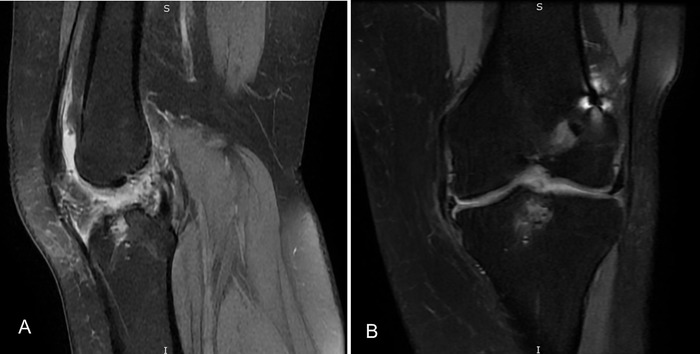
Magnetic resonance imaging of the left knee in (A) sagittal view shows complete tear of the anterior cruciate ligament (ACL) and (B) coronal view shows femur and tibia tunnels of previous ACL reconstruction with complete ACL tear and an absent patella.
